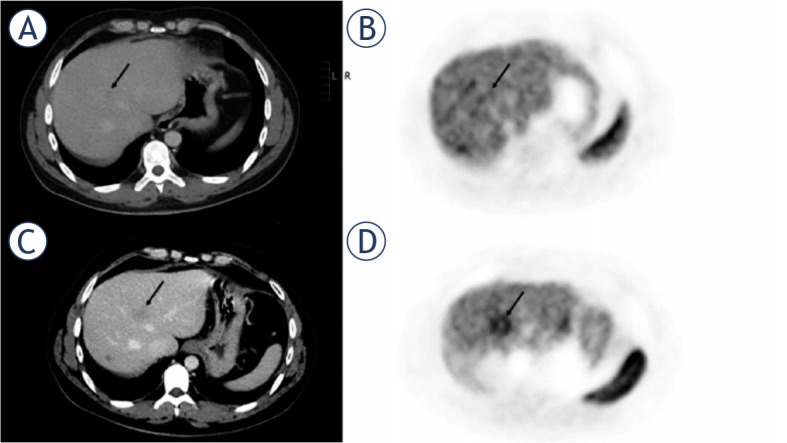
31-year-old male with non-responding liver metastasis of pancreatic neuroendocrine tumor. On the pretherapeutic PET/CT (A, B) there were low tumor-to-liver (T/L) ratios. After three months of treatment with everolimus, liver metastasis (arrow) showed an increase in size on CT (C), but also a significantly increased uptake of ^68^Ga-DOTATATE (D) compared to pretherapeutic PET/CT.
